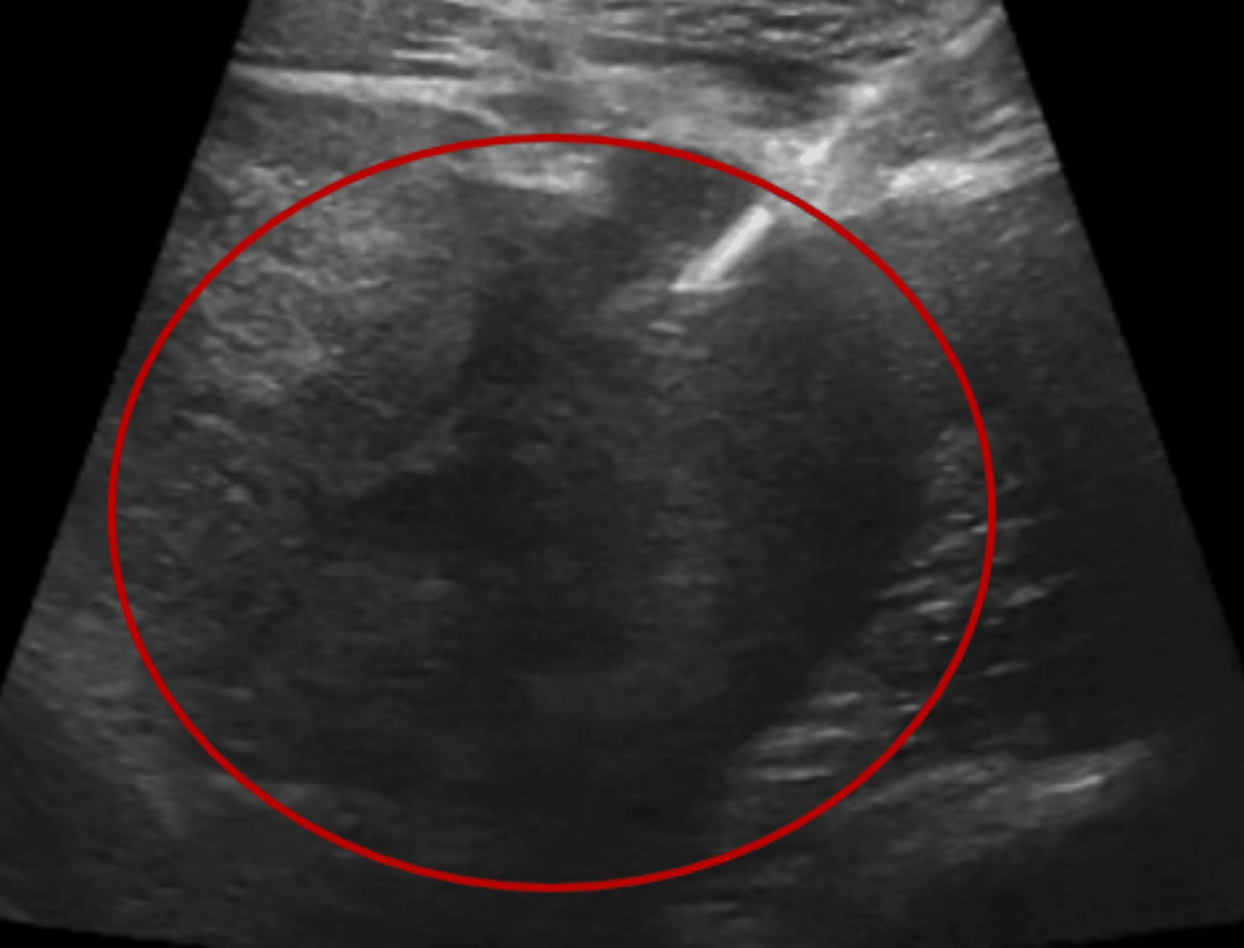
Abdominal ultrasonography revealing a large retroperitoneal mass.
